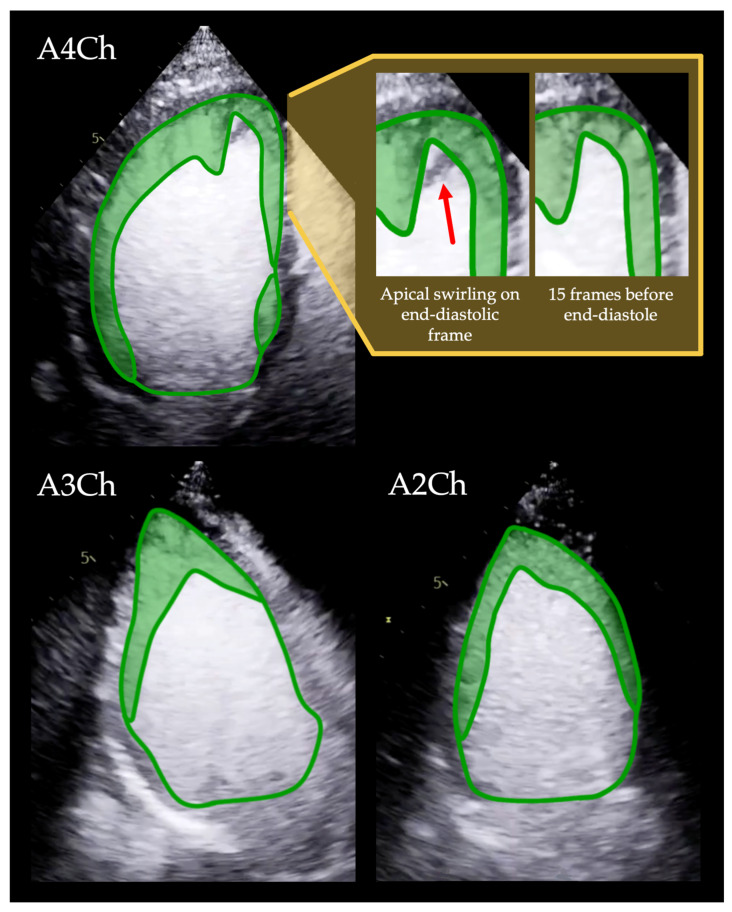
Trab_area tracing method using CE-Echo. The green lines show the Trab_area and LV_area tracings in the apical 4-3-2 chamber views, with the trabeculated area highlighted in light green. Before the yellow background, the apex is shown in the apical four-chamber view. The red arrow indicates an apical swirling artefact visible on the end-diastolic frame, which closely resembles trabeculation on the still image. However, it is not present 15 frames before the end-diastolic frame, confirming that it is an artefact rather than true trabeculation. Video S1. demonstrated the same cine loop, where the artefact’s transient appearance is clearly identifiable. This underscores the importance of assessing trabecular patterns in moving cine loops rather than relying solely on static frames. The video material for the same tracings, the apical 3 and 2-chamber views, can be seen in Videos S2 and S3, respectively. Note that in the 4-chamber view, two unconnected trabeculated regions are traced separately and combined to obtain the overall Trab_area in that view. Abbreviations: A4-3-2Ch: apical 4-3-2 chamber views; CE-Echo: contrast-enhanced echocardiography; LV_area: the area of the left ventricle; Trab_area: the area of the trabeculated layer.
